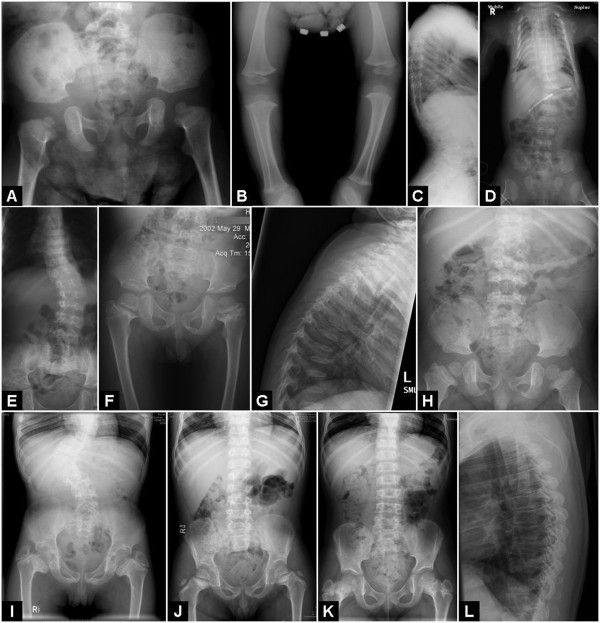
X-rays of some of the cases described. A, B, C, pelvis, legs and lateral spine of case 5 (MD-SMDK bridging phenotype); D, case 8 (MD); E, F, AP spine and pelvis of case 16 (SMDK); G, H, lateral spine and pelvis of patient 18 (SMDK); I, AP spine and pelvis of case 19 (SMDK); J, AP spine and pelvis of case 20 (SMDK); K, AP spine and pelvis of case 21 (SMDK); L, lateral spine and pelvis of case 26, who had some minor spinal changes and no metaphyseal involvement.
